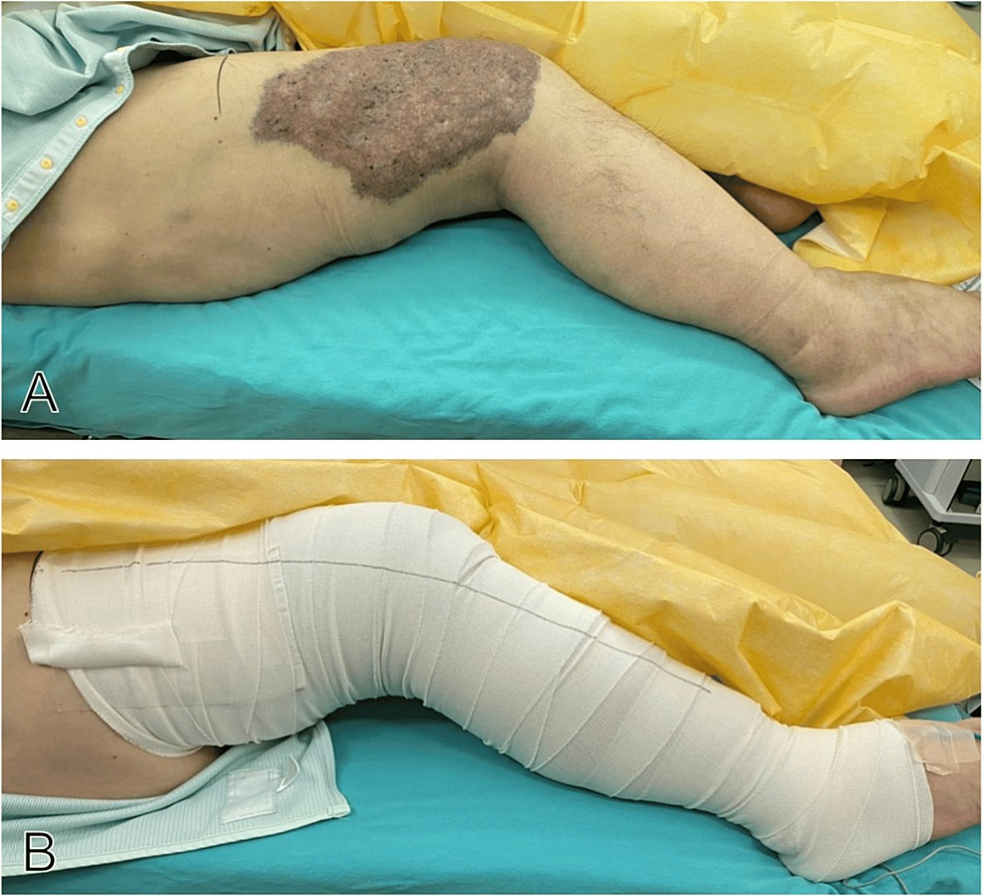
Elastic band compression (EBC) application A: Before EBC, B: After EBC. The femoral circumference from the superior border of the patella to the four lateral phalanges was 53.0 cm on the right (affected side) and 39.5 cm on the left (healthy side) before EBC. In B, the LDF sensor can be seen affixed to the dorsum of the foot. LDF – Laser Doppler flowmetry.
